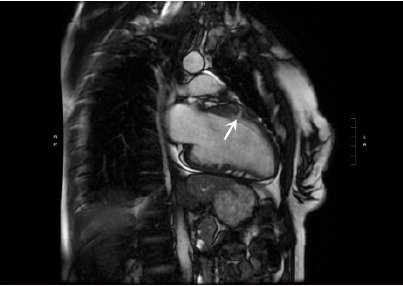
Cardiac magnetic resonance imaging confirming myocardial crypt within the anteroseptal wall (arrow). On final impression, there were two additional myocardial crypts noted within the inferoseptal wall. Otherwise, there was normal left ventricle and right ventricle wall thickness, wall motion, and ejection fraction.
